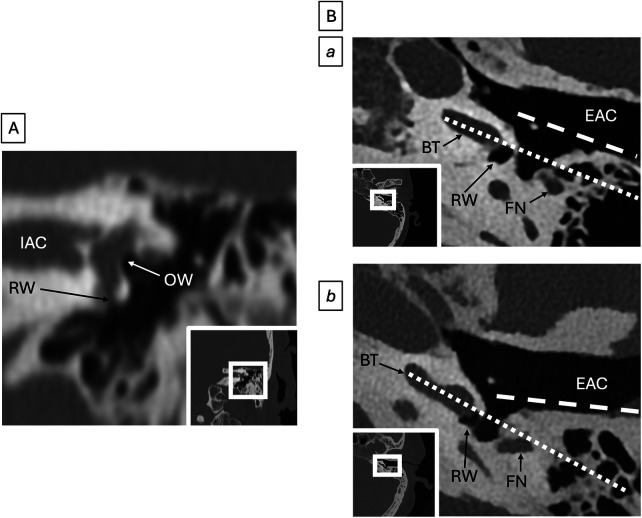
Round window anatomy (all images represent different patients). (A) Round window stenosis. Coronal CT in this patient with otosclerosis shows bony overgrowth causing severe stenosis of the round window (RW). Oval window (OW) and internal auditory canal (IAC) also shown for reference. (B) Photon-counting axial CT in patient (a) and patient (b) show a more favorable surgical approach in (a) compared to (b), as the long axis (dotted line) of the basal turn of the cochlea (BT) in patient (a) is parallel to the long axis (dashed line) of the posterior wall of the external auditory canal (EAC). Round window (RW) and mastoid facial nerve canal (FN) are provided for reference.
